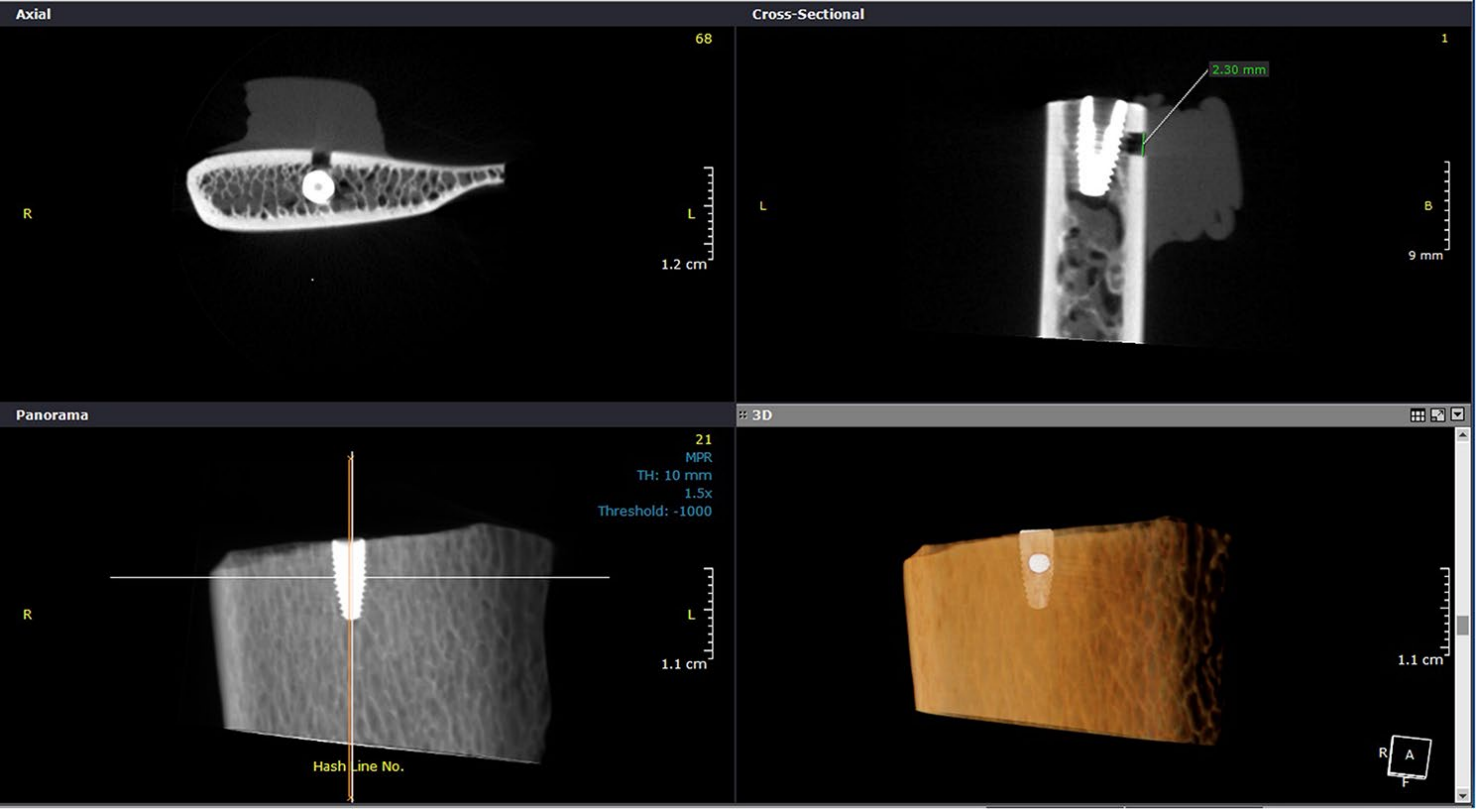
Measurement of fenestration diameter in CBCT images
